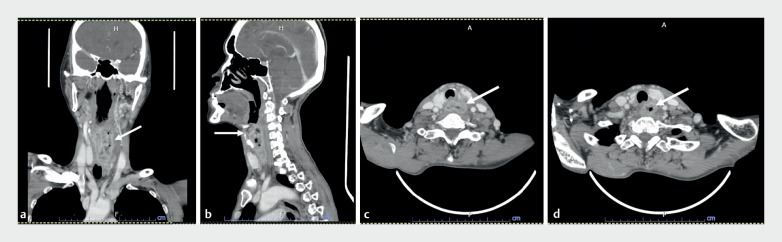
Computed tomography (CT) of the neck in a 54-year-old woman demonstrated an irregular circumferential enhancing mass involving the cervical esophagus, left epiglottis, left aryepiglottic fold, and the left pyriform sinus, with multiple cervical and paraesophageal lymph nodes (arrow):
